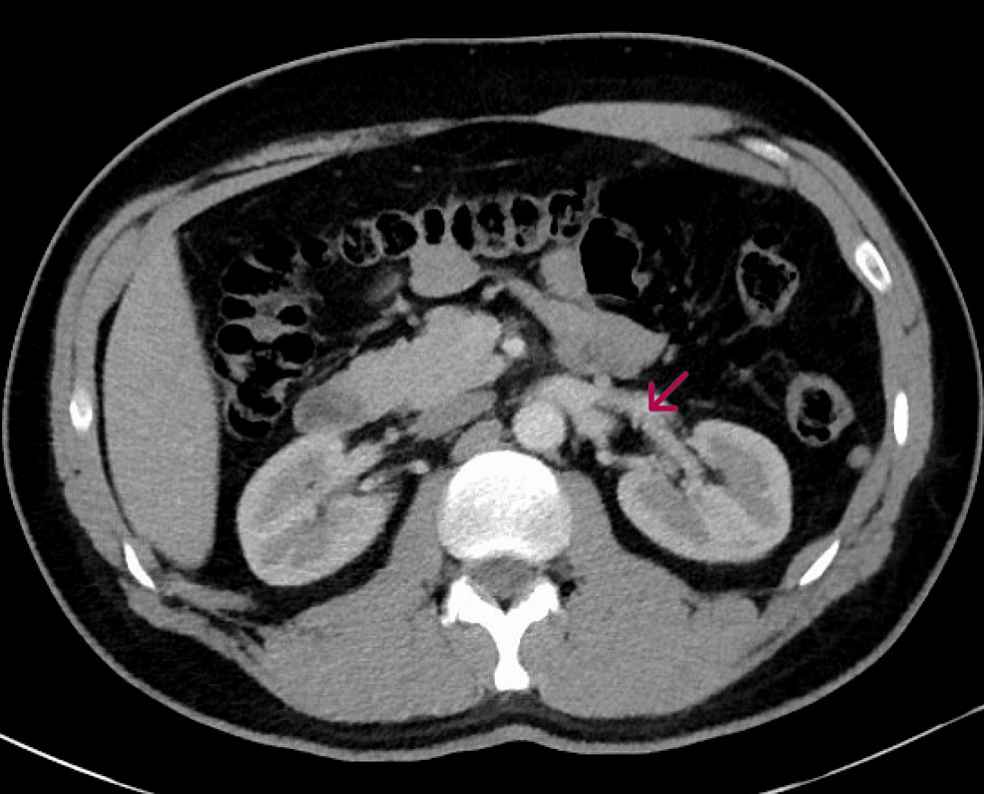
Computed tomography (CT) of abdomen and pelvis with contrast showing resolution of thrombus in the left renal vein after six months of treatment with a DOAC (red arrow) DOAC: direct-acting oral anticoagulant
